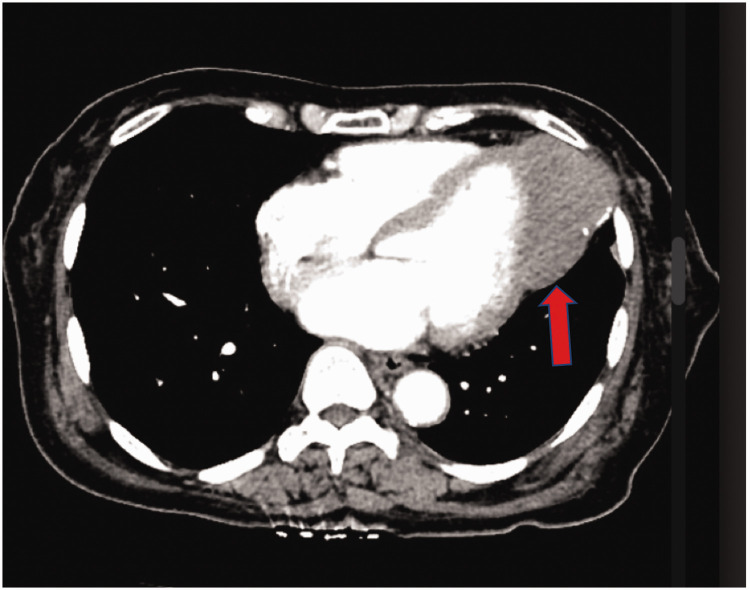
Axial cardiac computed tomography image showing large isodensity in left myocardial masses with calcifications, which suggests cardiac fibroma (red arrow) (used with permission).
